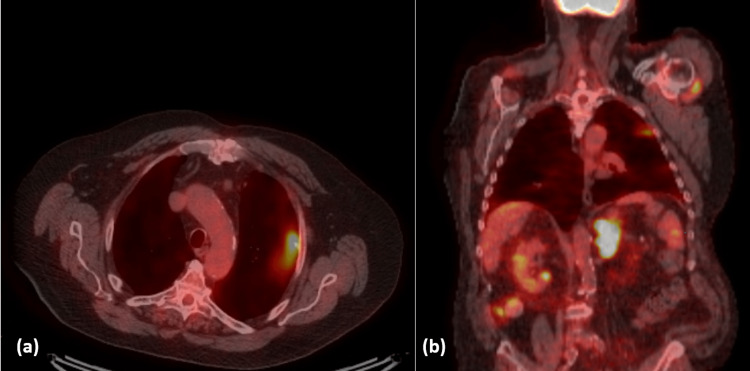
(a): Moderate to intense FDG activity can be seen fusing to left lung upper lobe peripheral reticulonodular changes, measured by SUV max 4.6. (b): Additional intense FDG activity fusing to the left renal mass, measuring SUV max 16.2, can be visualized along with the previously mentioned FDG activity seen in the left lung upper lobe.
